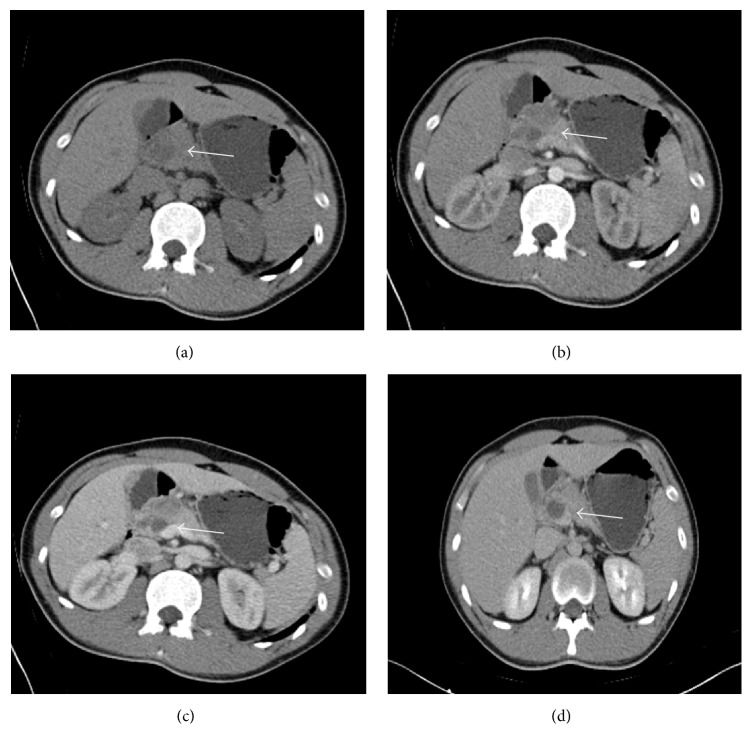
The CT scan revealed that the enlargement of the head of pancreas had an irregular density and unclear boundary. Moreover, inhomogeneous enhancement and progressive enhancement appeared after using iodinated contrast material. (a) CT scan, (b) arterial phase, (c) portal phase, and (d) delayed phase image.
